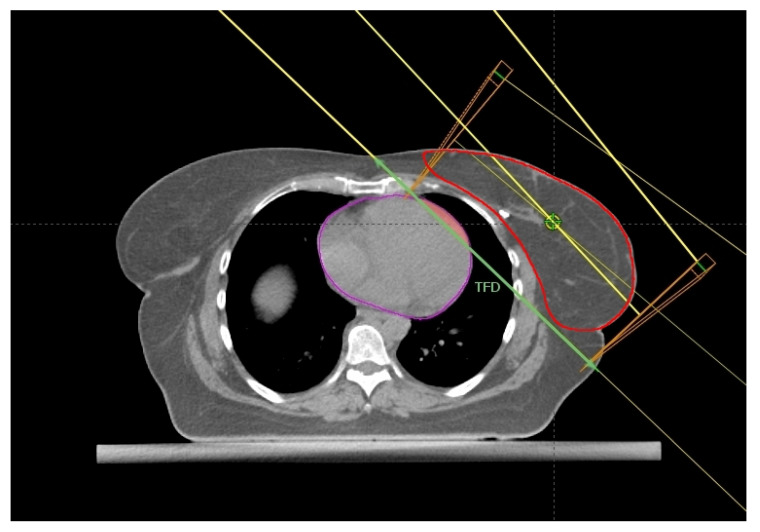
Axial CT plane passing through the liver dome with a standard tangential beam arrangement. The tangential field distance (TFD, green line), HVIF (pink area), and the CTV (red) have been highlighted.
